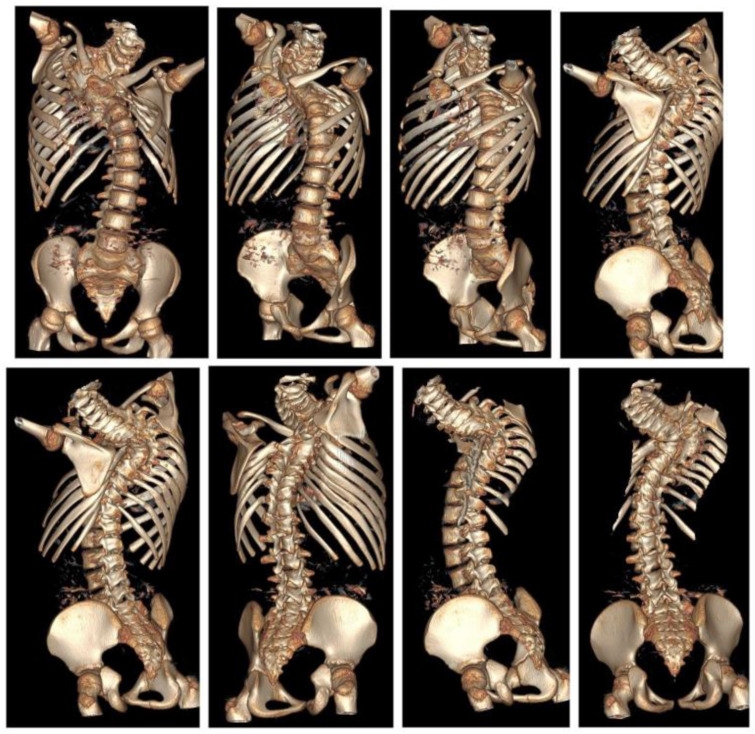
3D computed tomography of the five-year-old girl before surgical treatment.
